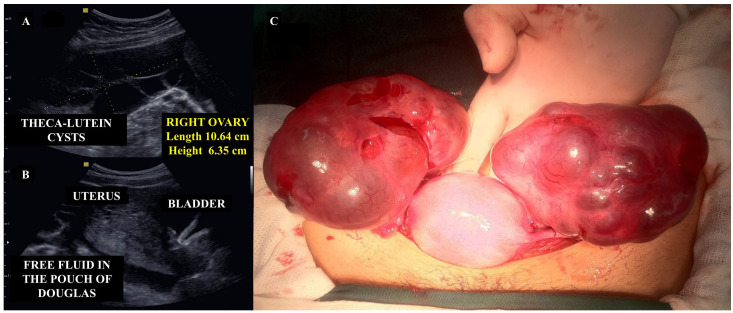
Imaging and surgical findings associated with theca lutein cysts. Panel (A): a pelvic ultrasound reveals a theca lutein cyst in the right ovary with a characteristic multicystic appearance. This case involves a patient at 13 weeks of gestation, with a pre-evacuation hCG level of 451,790 IU/L. Panel (B) shows a molar uterus accompanied by a large volume of free fluid in the pouch of Douglas, in a patient presenting with an acute hemorrhagic abdomen. There was rupture of the theca lutein cysts, resulting in hemoperitoneum. Panel (C) demonstrates the intraoperative findings from laparotomy consisting of theca lutein cysts with signs of rupture and active bleeding. The theca lutein cysts were aspirated, and chromic catgut 0 with a blunt needle was used for hemostatic suturing. Delicate surgical maneuvers were employed due to the extreme friability of this tissue. The images refer to clinical cases managed by the authors.
